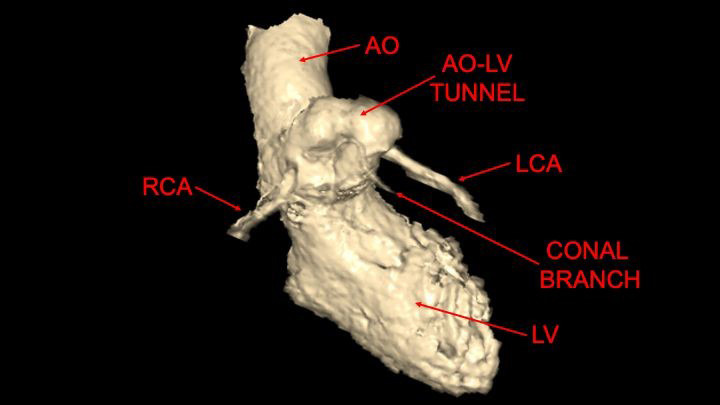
Three dimensional computed tomography reconstruction. This
